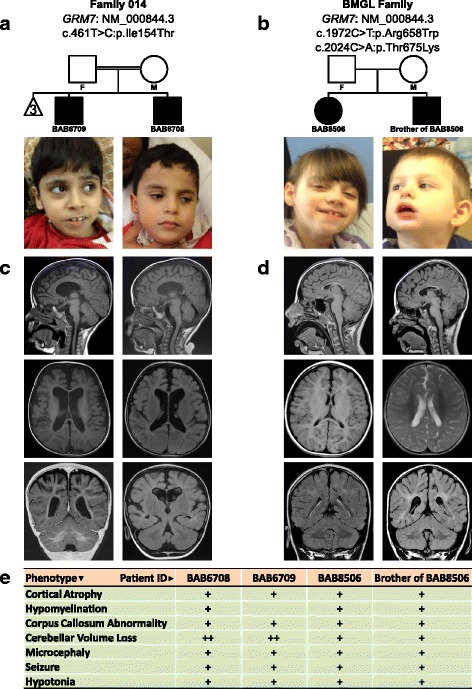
Comparison of the two families carrying *GRM7* variants. a Homozygous variant in *GRM7* (NM_000844.3:c.461 T > C:p.Ile154Thr) identified in family 014 with two affected children. b Compound heterozygous variants in *GRM7* (NM_000844.3:c.1972C > T:p.Arg658Trp and NM_000844.3:c.2024C > A:p.Thr675Lys) segregates in the family with two affected children identified in the BMGL database. c MRI images of BAB6708 and BAB6709. d MRI images of BAB8506 and her brother. e Clinical features comparison among four patients
